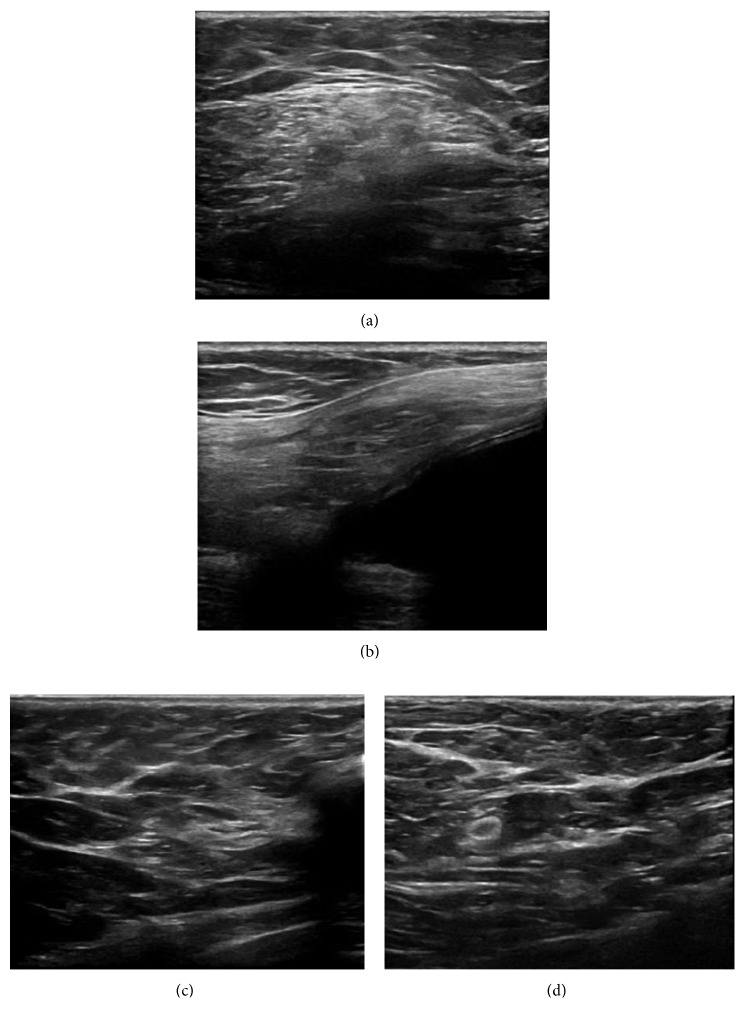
Ultrasound of the left breast ((a) transverse view, (b) sagittal view at 12 : 00 position) and the right breast ((c) transverse view, (d) sagittal view at 10 : 00 position).
